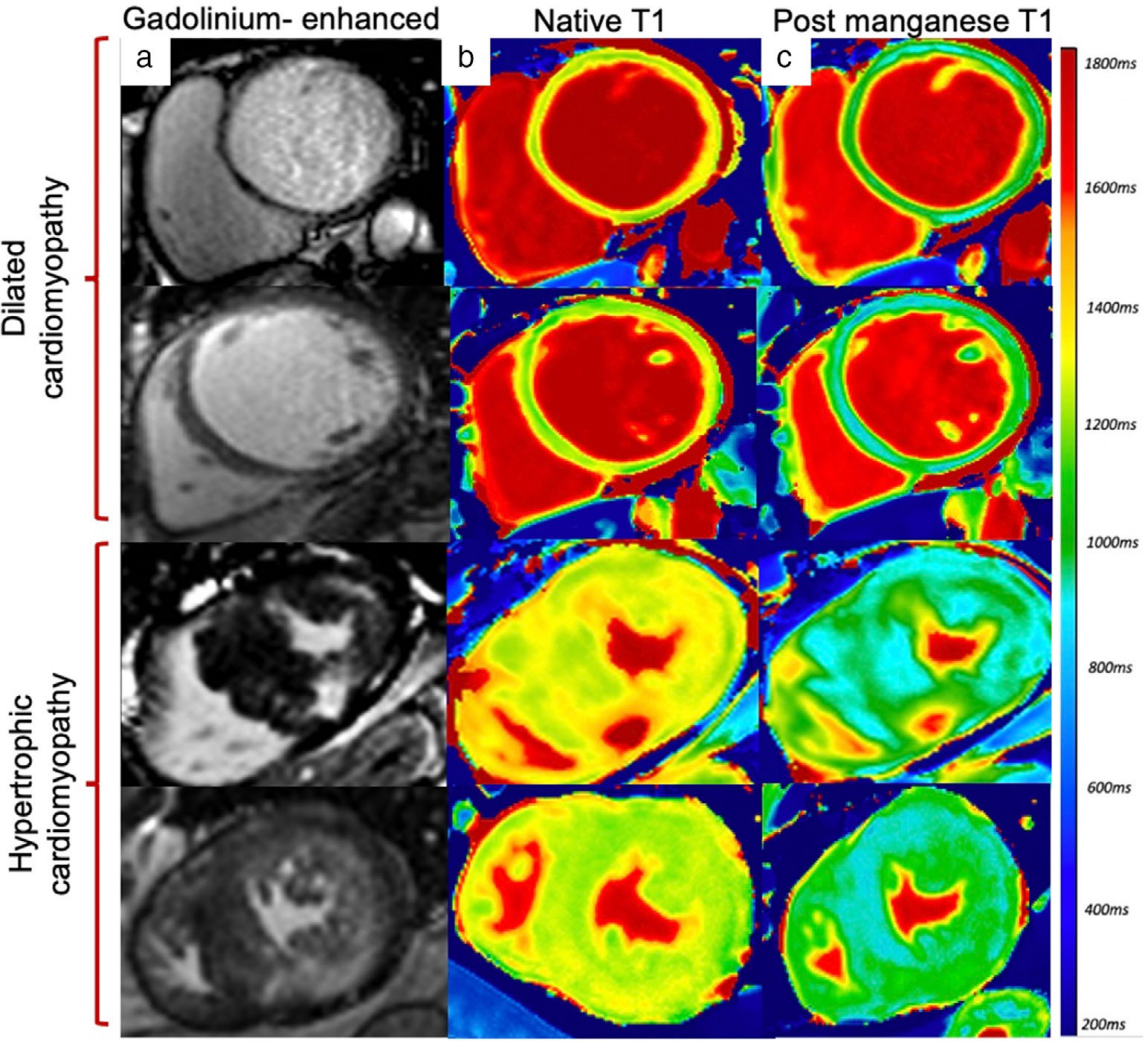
Manganese‐enhanced magnetic resonance imaging in ischemic and nonischemic Cardiomyopathy. Short‐axis views of gadolinium‐enhanced images (a), native T1 (b) and 30‐minute post‐manganese T1 maps (c) in patients with dilated and hypertrophic cardiomyopathy.
